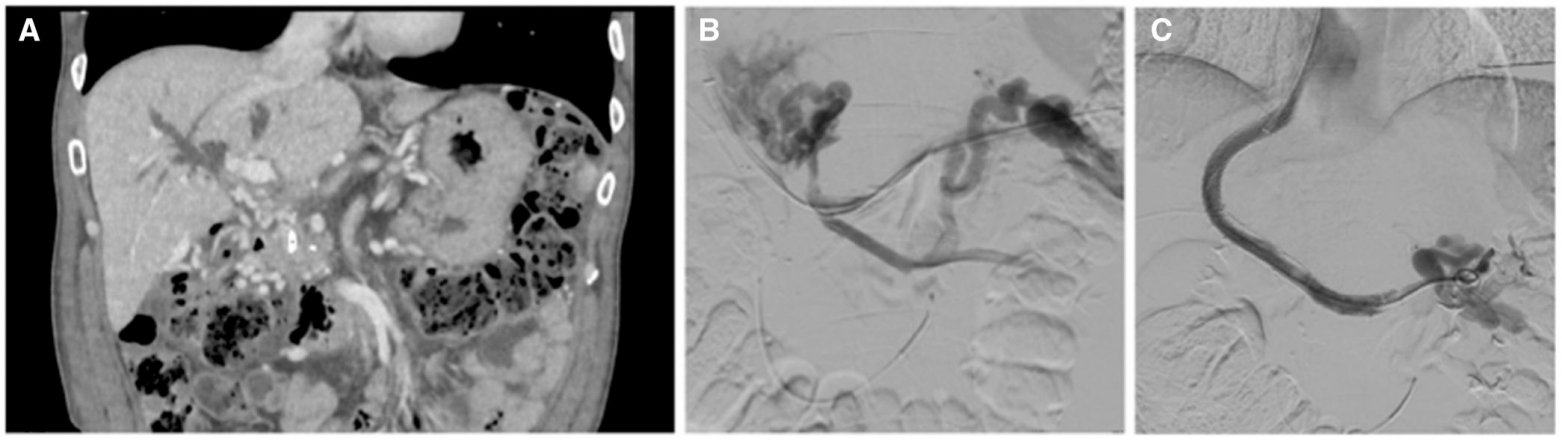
(A) Contrast enhanced CT scan, venous phase, showing cavernomatous transformation of extra-hepatic portal vein and portal biliopathy. (B) Catheterization of the native portal vein by a combined percutaneous trans-jugular intra-hepatic and trans-splenic approach. (C) Final phlebography at TIPS positioning.
